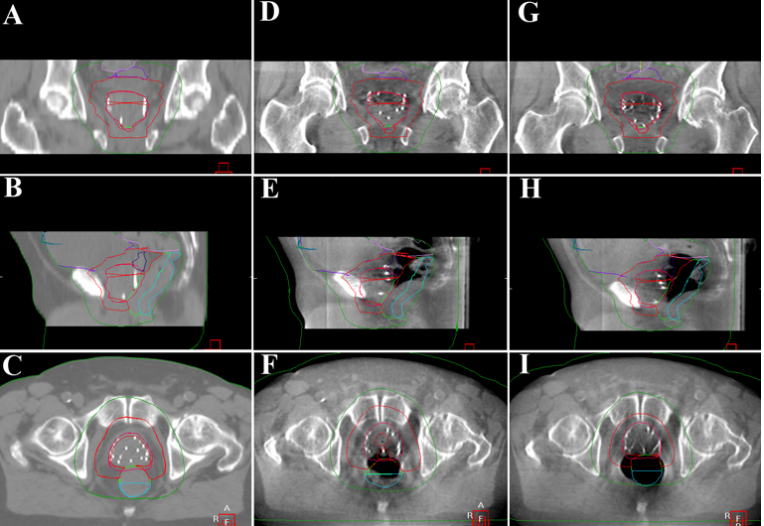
Planning CT (A-C) and CBCT images of a patient immediately before (D-F) and after (G-I) an IMRT fraction. Prostate position differs due to differences in rectal filling during therapy caused by rectal gas not present on treatment planning (in this case -7.4 mm in AP, 1.1 mm in left-right and -1.0 mm in cranio-caudal directions). Planning CT was acquired due to the intitutional policy using enema. This patient marks the maximum unidirectional displacement observed. In general patients with larger differences in rectal filling between treatment planning CT and daily online cone beam CT are asked to empty their rectum and drink water before another treatment attempt.
